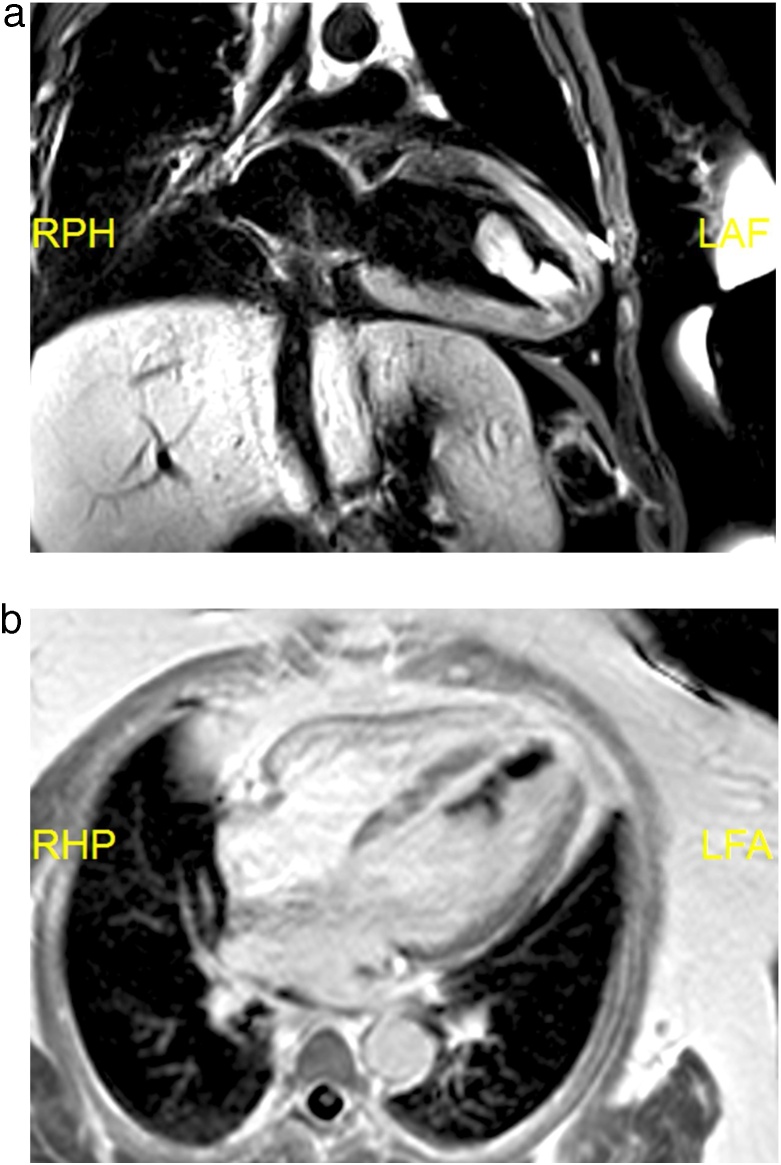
a -b. Cardiac magnetic resonance imaging of left ventricular clot. a Fat-saturated T2 weighted magnetic resonance imaging. b Fixed delayed T1 weighted magnetic resonance imaging at 600 milliseconds showed the mass is dark. This is specific for thrombus.
